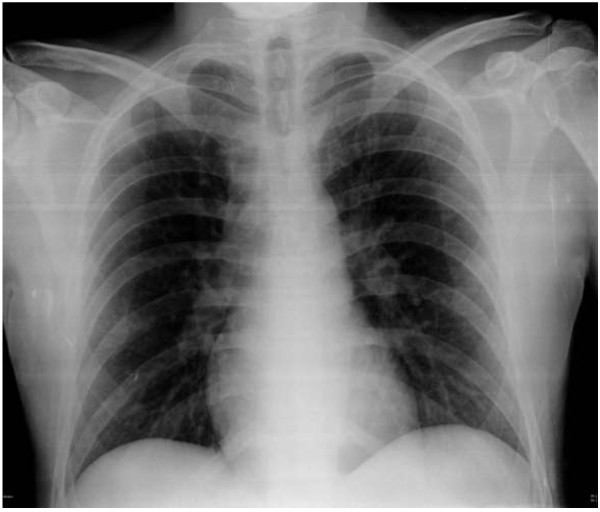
Normal preoperative anteroposterior chest radiograph.
